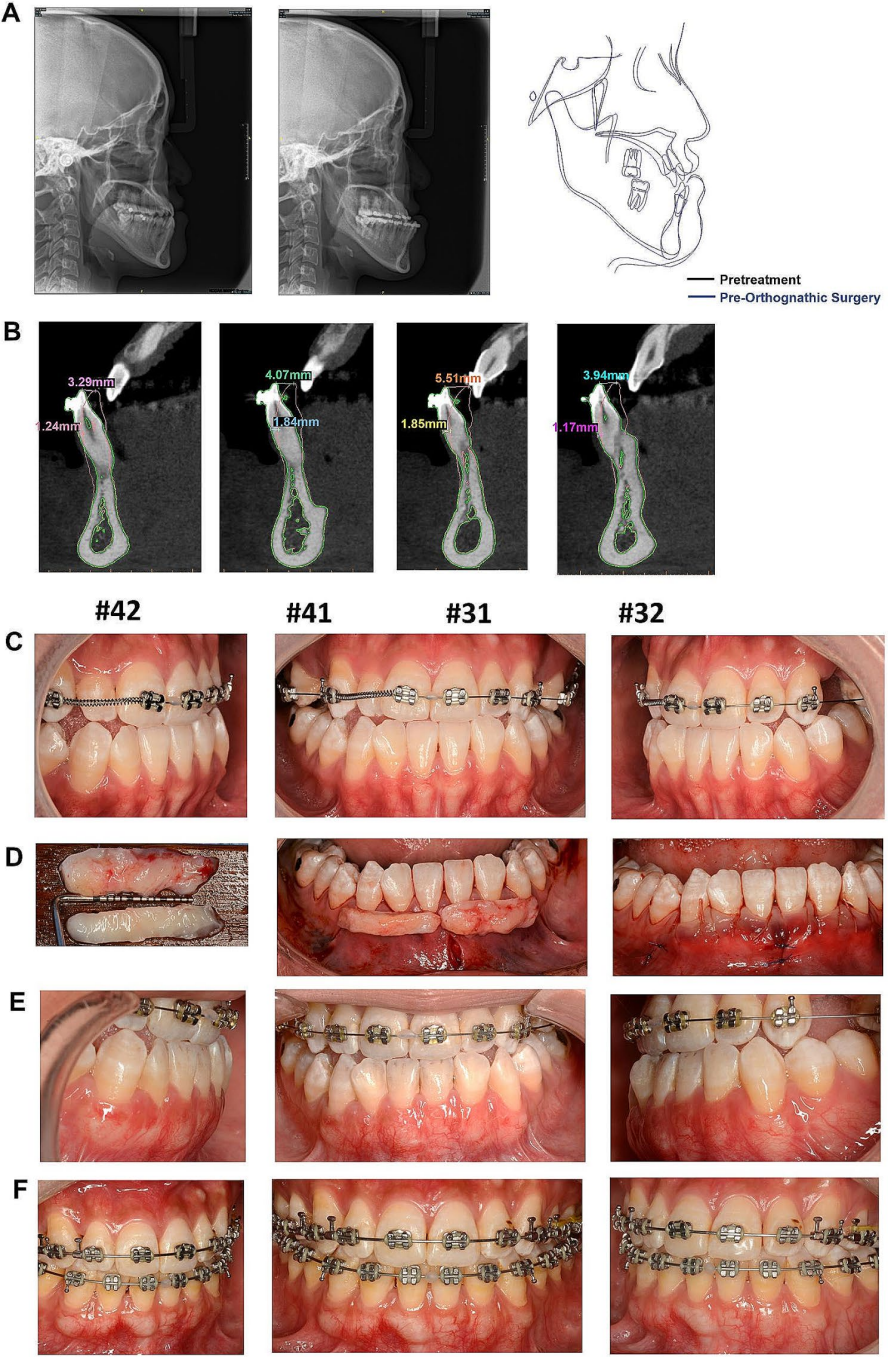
Cephalometric radiographs, CBCT and clinical photographs of case 1. A The comparison of the cephalometric radiographs of the baseline and the end of pre orthognathic surgical orthodontic treatment. B The superimposition images of the #31#32#41#42 CBCT data at baseline and at the end of pre orthognathic surgical orthodontic treatment. C The appearance of the anterior mandibular teeth with thin periodontal phenotype at baseline. D Subperiosteal tunnel was extended from the vestibular to the gingival margin, which accommodated a 2 mm thick and at least 5 mm apico-coronal-dimensioned autogenous connective tissue graft. E The photographs at three months after the PhMT-s surgery. F The photographs at the end of orthognathic surgery
